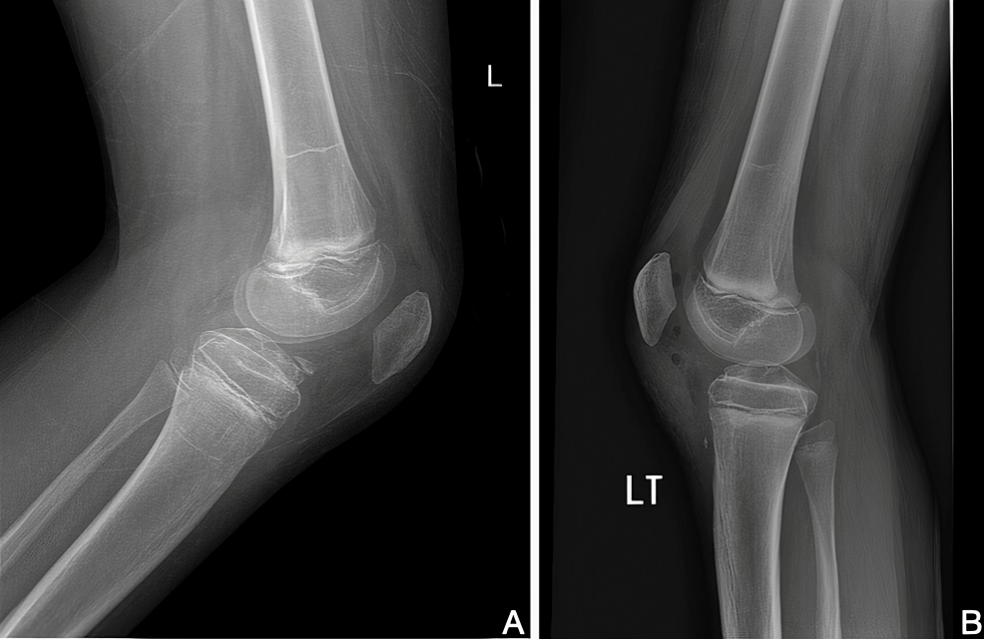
A: Showing a lateral X-ray of the left knee preoperatively reveals a partial bony avulsion of the tibial spine. B: Showing a lateral X-ray of the left knee postoperatively confirms the excision of the avulsed part.
